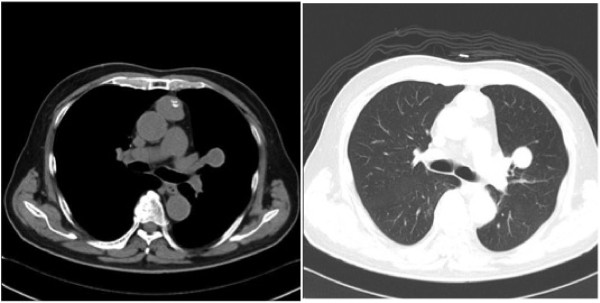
Computed tomography (CT) scan shows an anterior mediastinal mass with calcification, approximately 3 cm in diameter, and a 2 cm well-defined hilar nodule in the left upper lobe.
